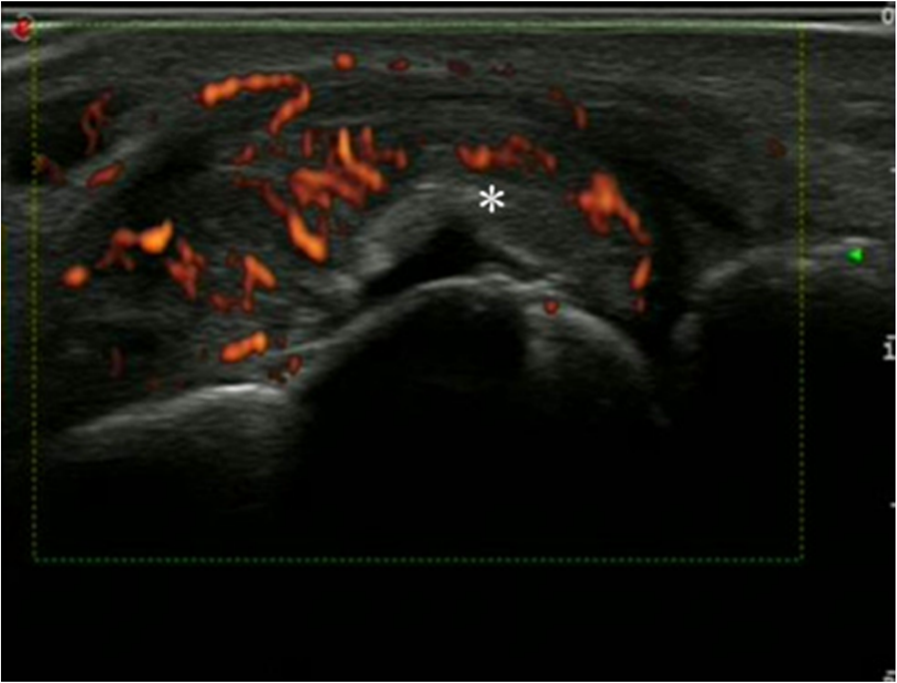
The ultrasound image for tophi in gout patients. The red signal represented the tophi in gout patients
